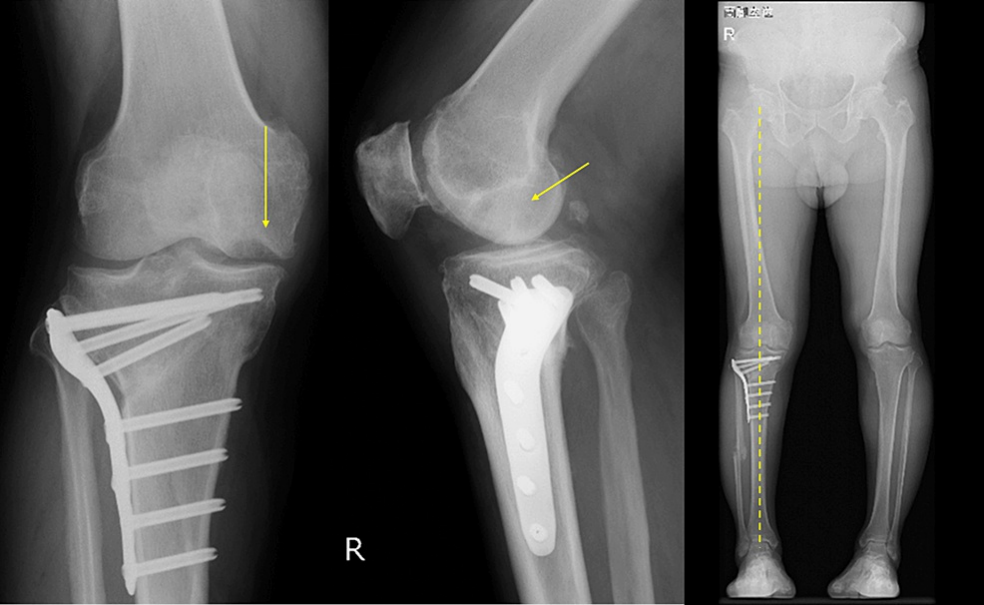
Radiograph of the right knee at one year postoperatively The arrows indicate bone and cartilage defects. The dashed line is the Mikulicz line.
